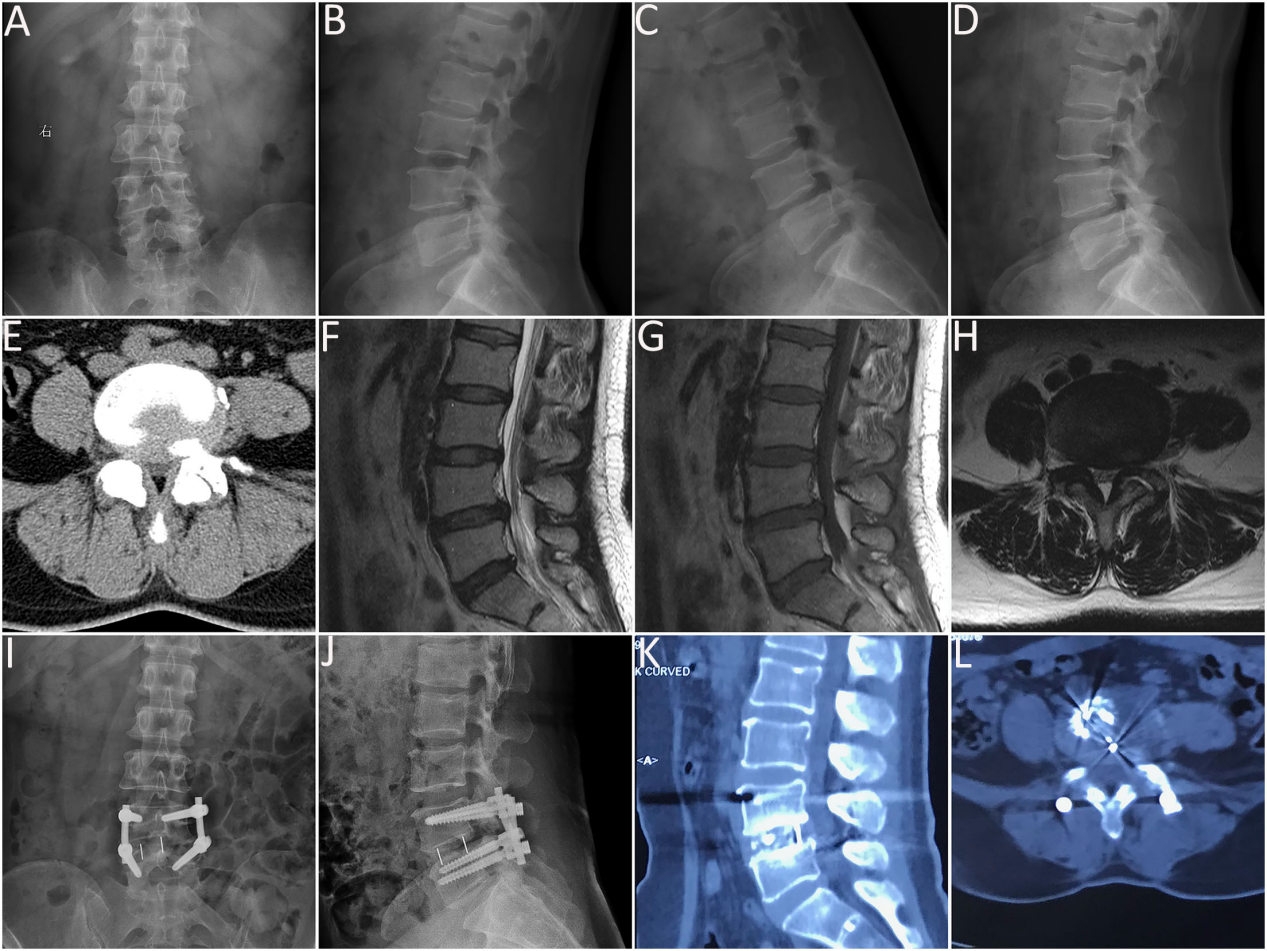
Minimally invasive transforaminal lumbar interbody fusion (MI-TLIF) performed on a 48-year-old female patient diagnosed with L4-L5 lumbar spinal stenosis with degenerative spondylolisthesis. (A,B) preoperative anteroposterior and lateral plain radiographs; (C,D) preoperative flexion and extension radiographs; (E) preoperative computed tomography (CT) scans; (F–H) preoperative magnetic resonance imaging (MRI) scans; (I,J) anteroposterior and lateral plain radiographs 3 days after the surgery; (K,L) CT scans 12 months after the surgery.
